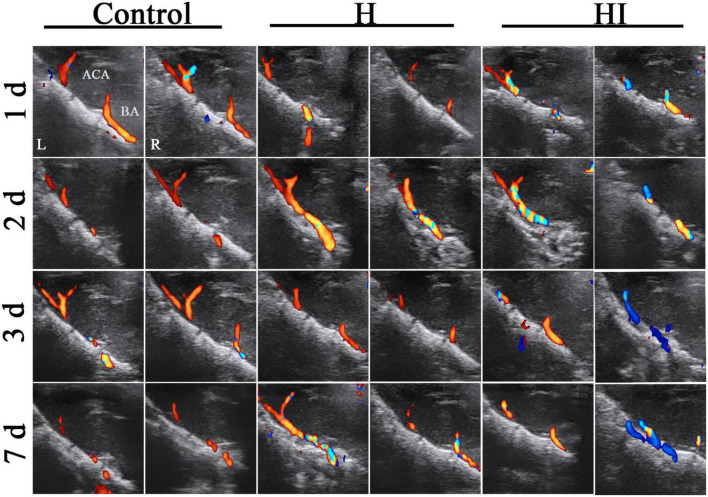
Sagittal dynamic acquisition of ultrasound detection of cerebral vessels. Left and right cerebral vessels in the sagittal position observed at 1, 2, 3, and 7 days after operations in the control (*n* = 7), H (*n* = 5), and HI (*n* = 5) groups. ACA, anterior cerebral artery; BA, basilar artery; H, hypoxia; HI, hypoxia and ischemia; d, days; L, left; R, right.
